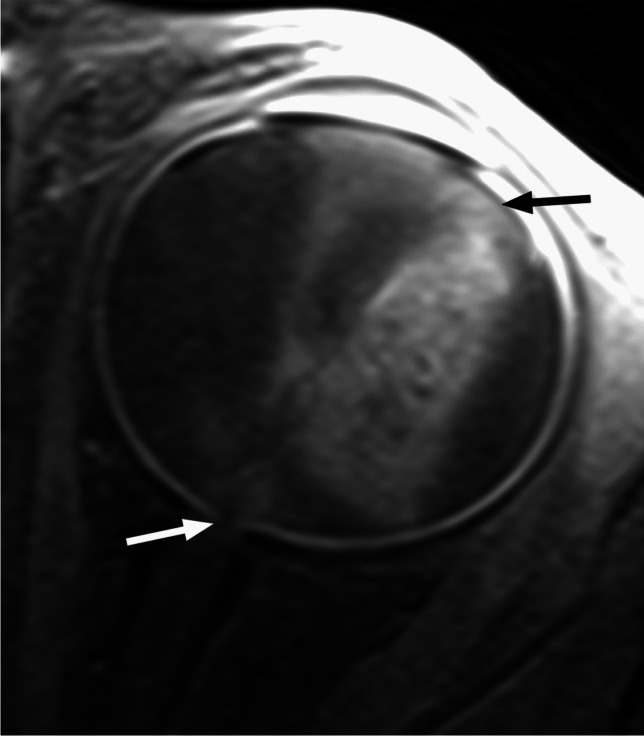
Axial postcontrast T1-weighted image in another patient with retinoblastoma shows optic nerve enhancement and interruption of the choroidal enhancement (white arrow), indicative of neoplastic invasion of the nerve. There is also extension of the enhancing mass to the anterior ocular chamber (black arrow)
